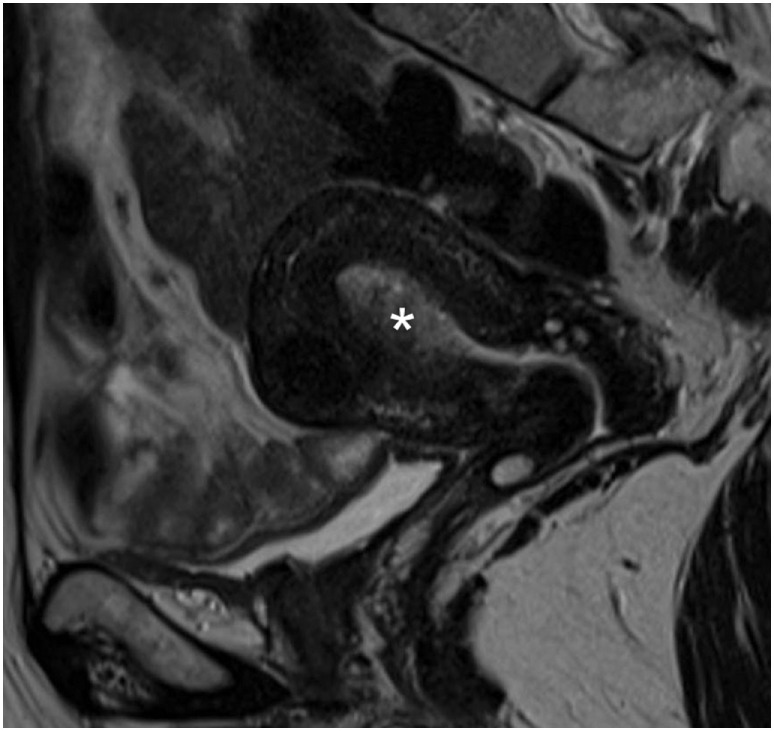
Pelvic magnetic resonance image. Sagittal T2-weighted magnetic resonance image showing endometrial cancer as heterogeneous intermediate-signal intensity lesion (white asterisk) disrupting the normal high-signal intensity endometrium and infiltrating less than half of the ventral myometrial thickness (revised FIGO IA Stage).
